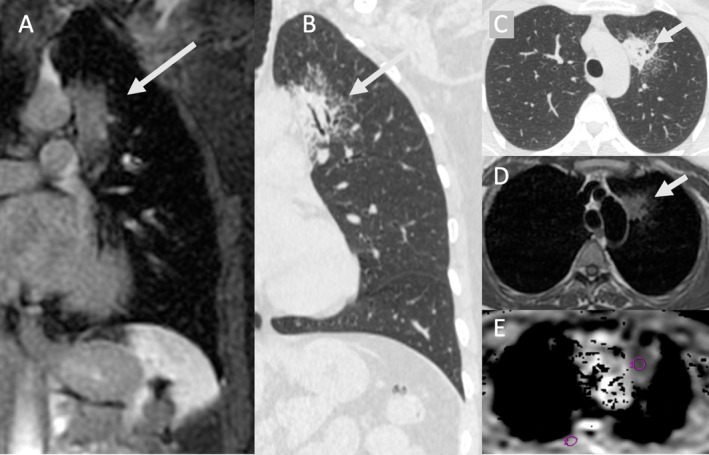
A 42‐year‐old female with a subsolid lesion and consolidation in the juxta‐mediastinal left upper lobe, final diagnosis of adenocarcinoma. A coronal T2‐weighted image with fat signal suppression (A) and a transverse T1‐weighted fast spin echo acquisition (D) show an unspecific, intermediate signal intensity. The ADC map from DWI shows diffusion restriction (E); transverse CT (C) and a coronal reconstruction from CT (B) for reference.
